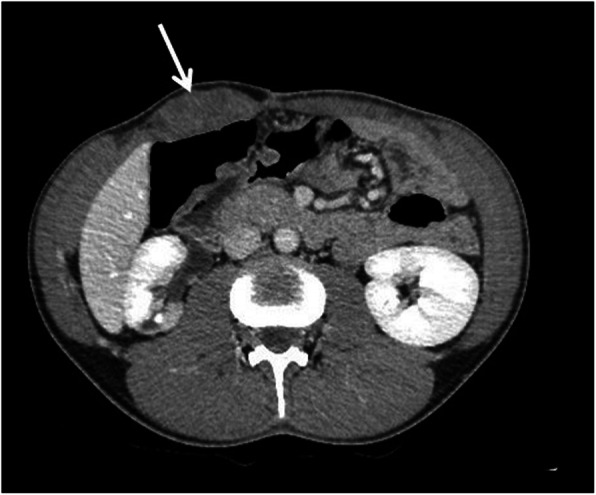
CT appearance of an abdominal wall DT (arrow) in a 26-year-old male patient affected by Gardner syndrome with previous total colectomy
